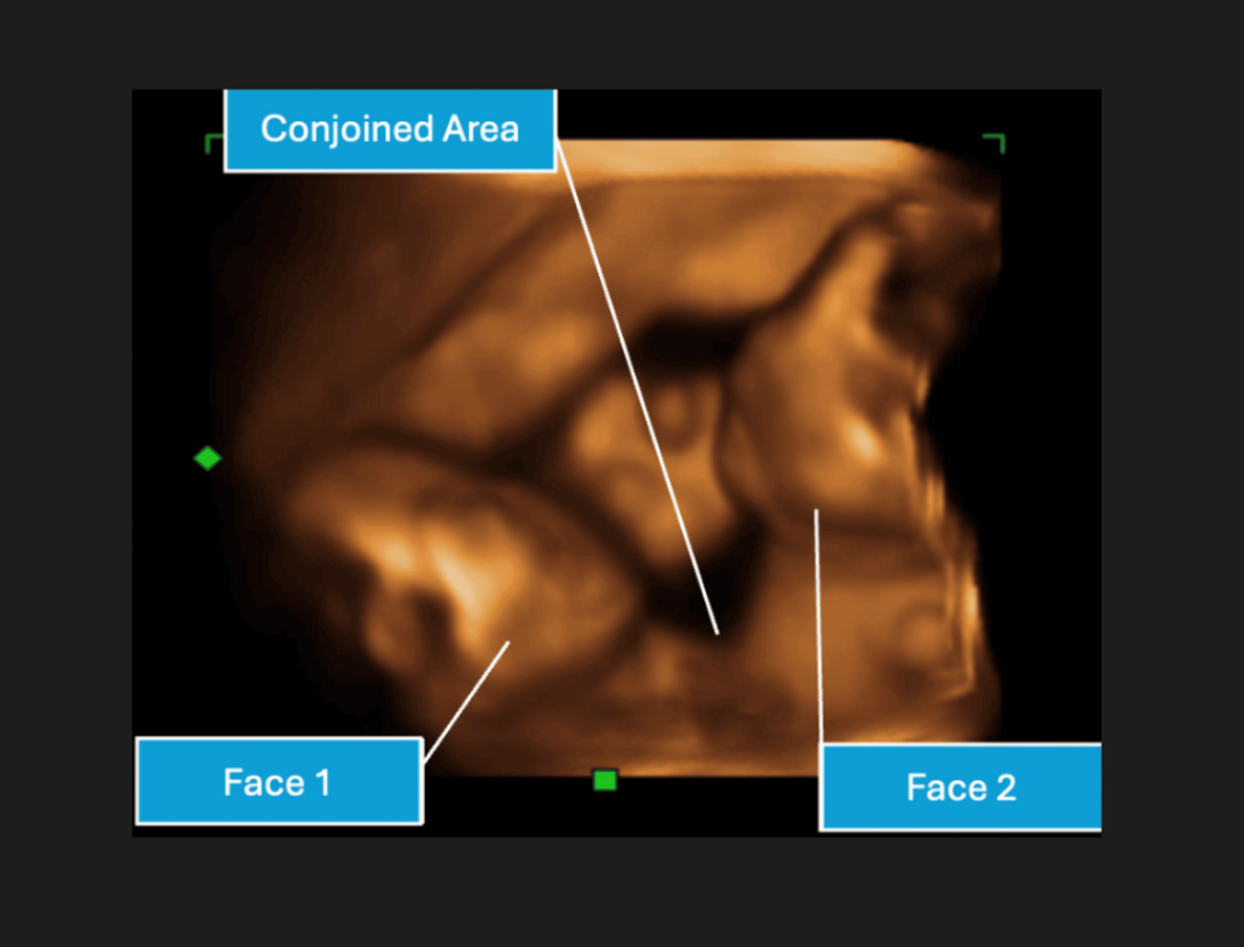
3D surface rendering mode showing a transverse view of 3D ultrasound. The blue labels depict the conjoined twins facing one another, with both faces labeled.
